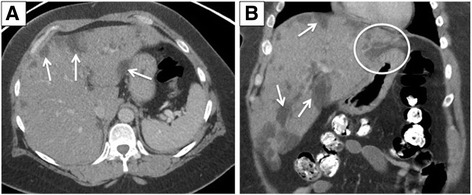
Contrast-enhanced computed tomography of the liver in (a) axial and (b) coronal views. Multiple hypodense lesions are noted throughout the liver in a subcapsular distribution (*arrows*) as well as perivascular (*circle*)
